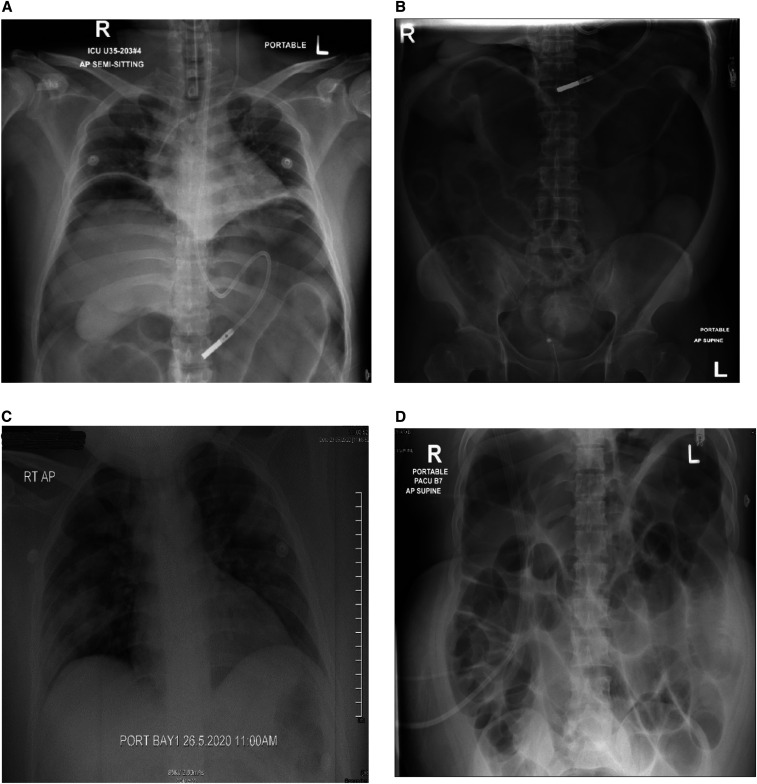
Radiographs for patients diagnosed with COVID-19 pneumonia. (A) Chest radiograph for case 1 showing bilateral free air under the diaphragm and (B) abdominal radiograph showing distended bowel loops. (C) Chest radiograph for case 2 showing bilateral patchy infiltrates and (D) abdominal radiograph showing diffuse dilatation of the small and large bowel loops.
